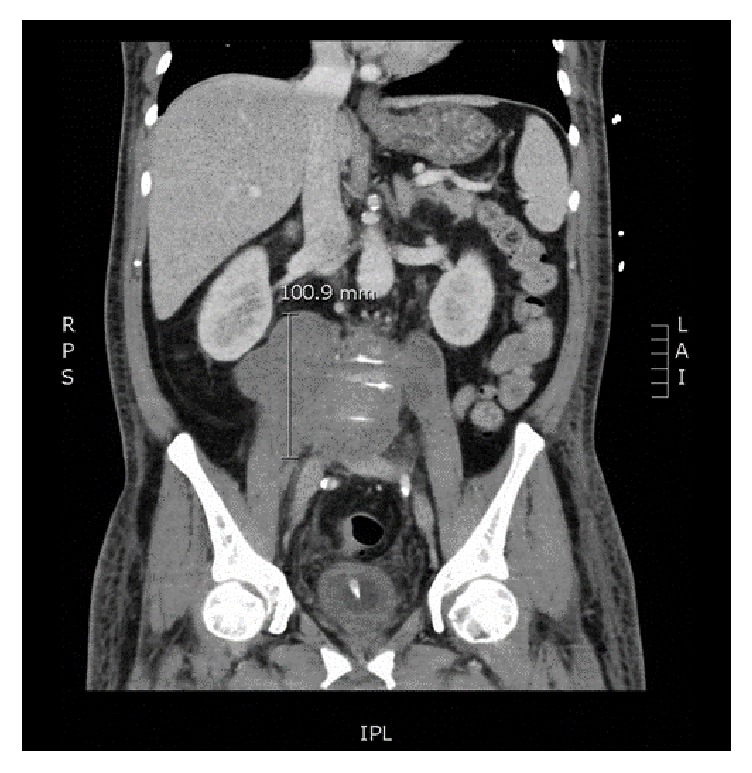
Abdominal CTA showing a lobulated low-density soft tissue mass within the retroperitoneum with involvement of the bilateral psoas muscles.
